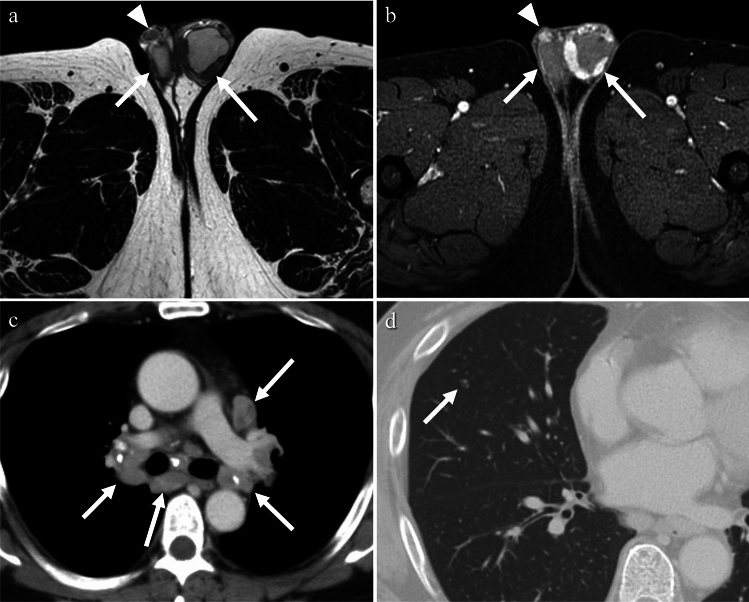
Man in his 40 s with sarcoidosis presenting with left inguinal tenderness. a Multiple nodules with low signal intensity are observed in both the testes (arrows) and epididymis (arrowhead) on T2-weighted images. b On fat-saturated contrast-enhanced T1-weighted images, the nodules exhibit strong enhancement (arrows and arrowhead). c, d A chest CT revealing calcified mediastinal and hilar lymphadenopathy (arrows in c) and centrilobular granular nodules (arrow in d). These findings led to a diagnosis of sarcoidosis
